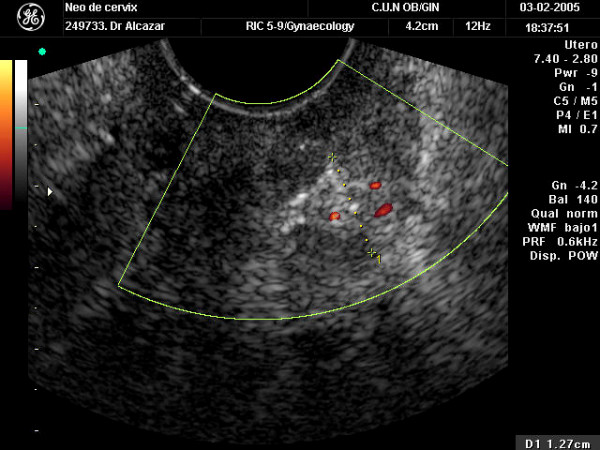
Transvaginal color Doppler ultrasound showing a cervical cancer with scanty vascularization.
